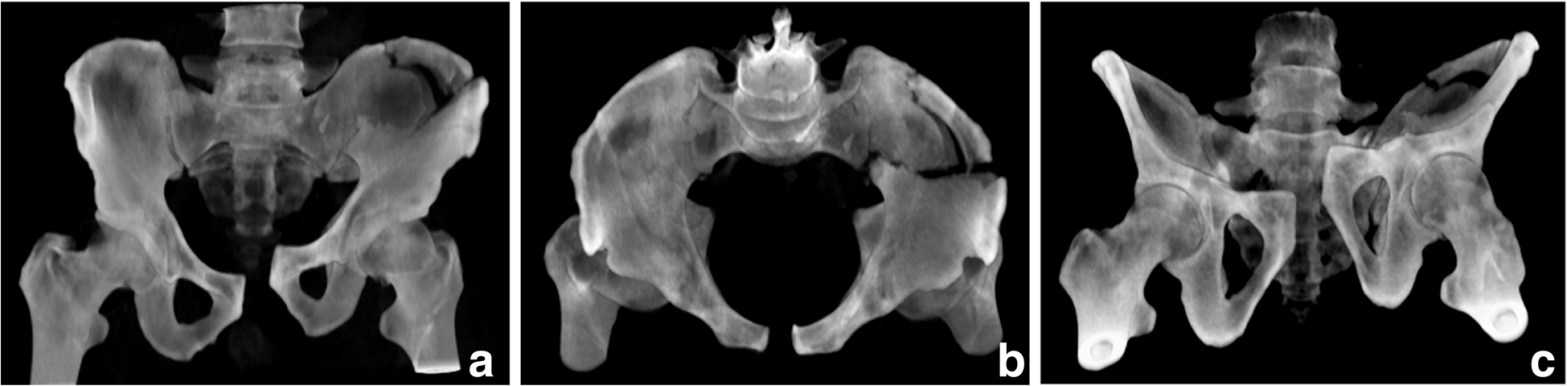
CT-generated AP (a), inlet (b) and outlet (c) pelvic radiographs of the patient from Fig. 1
